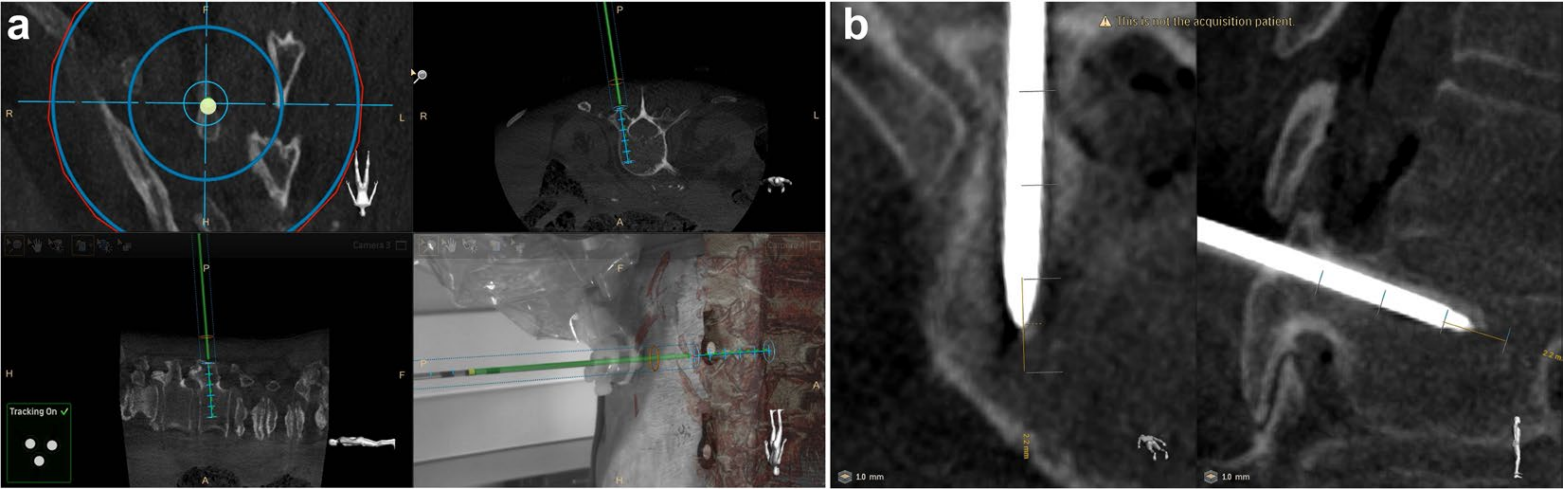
Depiction of the surgical navigation interface during robot assisted surgery: In (a), top left shows a coronal view along the pedicle trajectory, top right shows an axial view with the navigated Jamshidi in green, bottom left a sagittal view, and bottom right a camera feed with an augmented reality overlay depicting the underlying anatomy. In (b), a post-operative computed tomography scan of a placed Jamshidi needle is depicted. Axial view to the left and sagittal view to the right.
